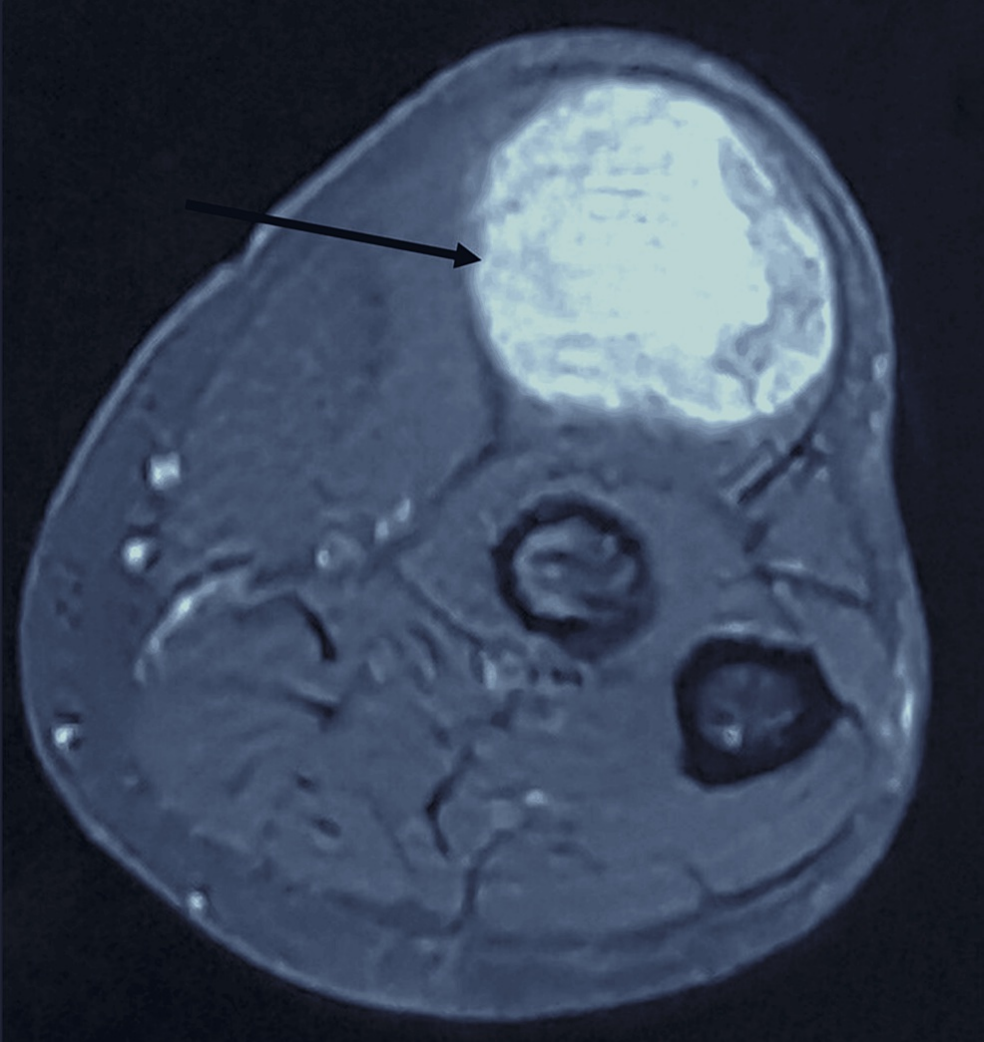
Axial proton density fat-saturated MRI view of the right forearm revealing a mass in the right forearm's posterolateral aspect
